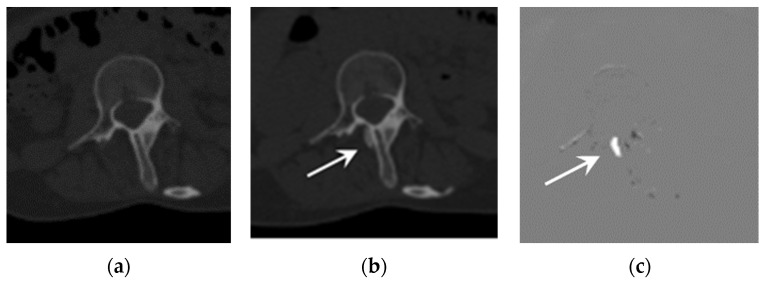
Example of an emerging lesion. CT images of a 25-year-old FOP patient: (a) previous CT image, (b) current CT image, and (c) TS (subtraction) image. The emerging lesion adjacent to the thoracic spine (arrow) is difficult to detect on the current CT image, but is clearly visible in the TS image.
